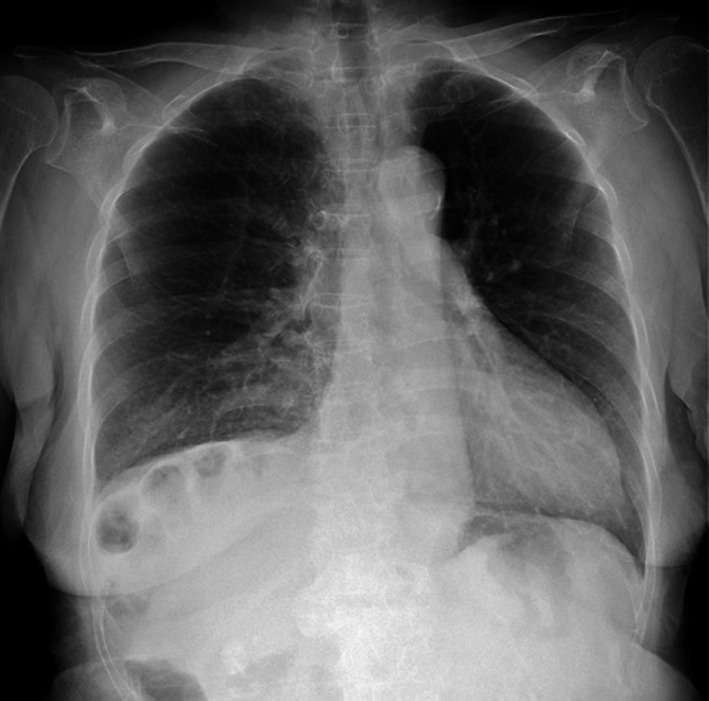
Chest radiography. Chest radiography reveals a massive abnormal intestinal gas above the right lobe of the liver
